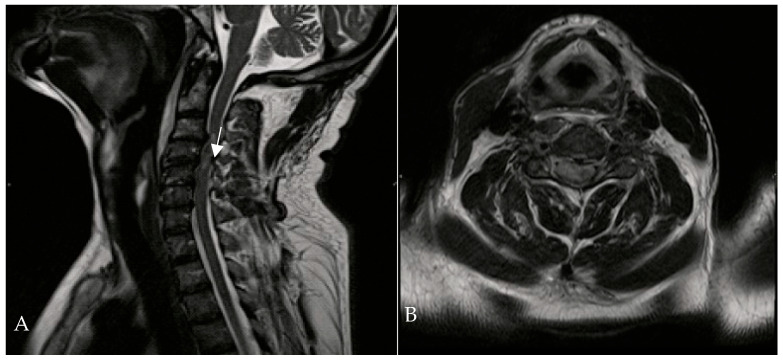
Cervical MRI—T1-weighted sagittal (A) and transverse planes (B)—showing hematomyelia (white arrow) at the C3-C4 level without vertebral fractures.
